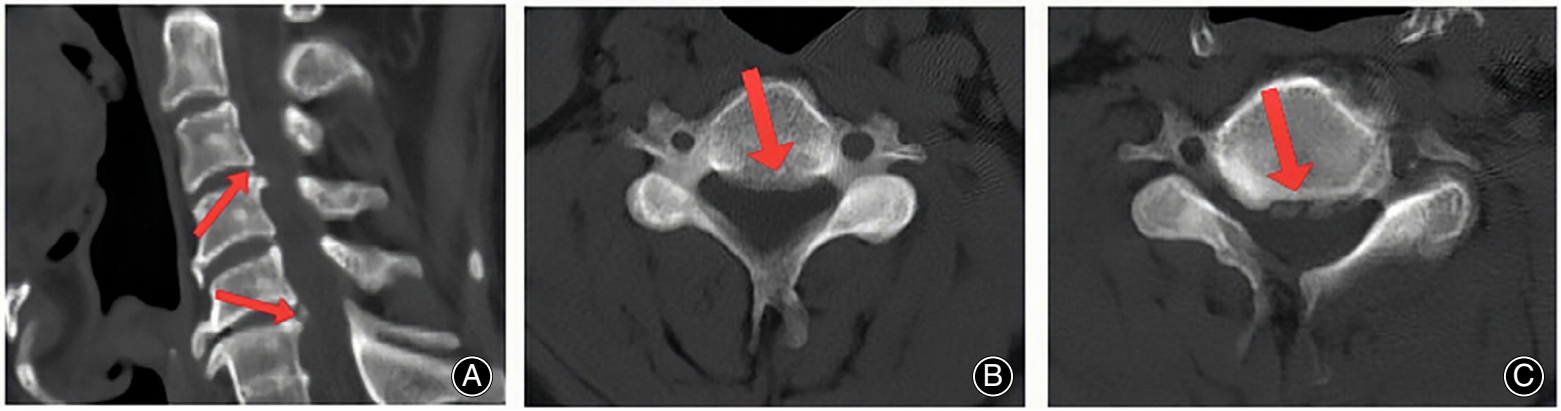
Preoperative CT scan. The sagittal section showed that the C3/4 and C5/6 level intervertebral disc‐osteophyte complex was formed (arrow) (A). The transverse section showed that the intervertebral disc‐osteophyte complex formed at the C3/4 level (arrow) (B) and C5/6 level (arrow) (C).
